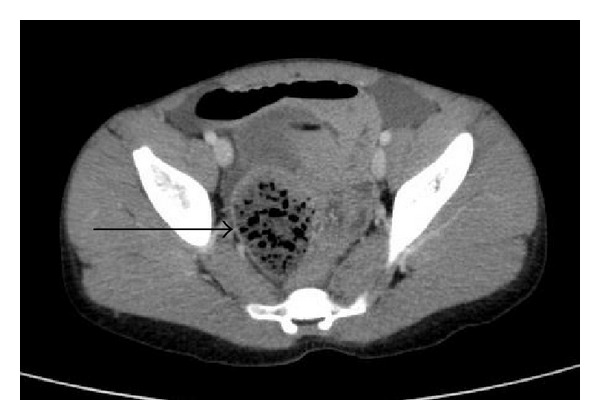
Axial CT images with oral contrast showing dilated loops of jejunum and proximal ileum. The rhubarb bezoar can be seen in the lumen of small bowel.
